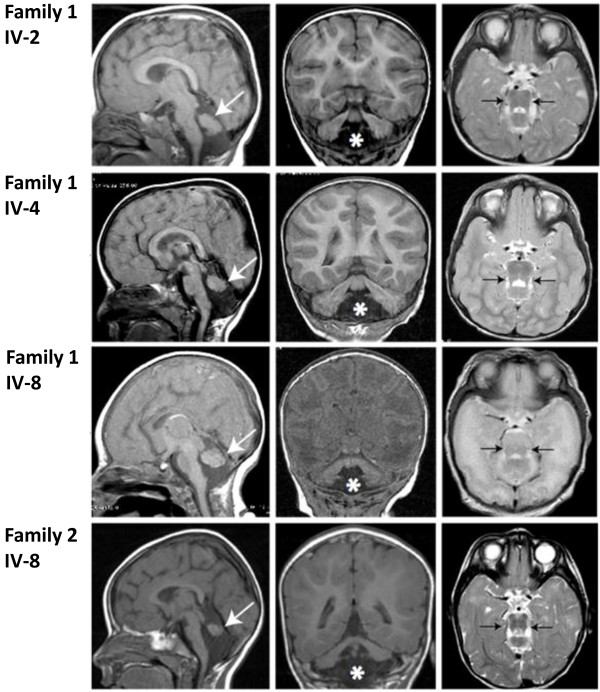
Brain MRI of affected individuals from Families 1 and 2. Left: midline parasaggital T1-weighted images showing absent inferior vermis, and hypoplastic inferior-cerebellum (arrows). Middle: coronal T1-weighted images showing neocortical pachygyria and mega cisterna magna (*). Right: Axial T2-weighted images showing typical square-shaped midbrain-hindbrain junction morphology in all patients (arrows).
